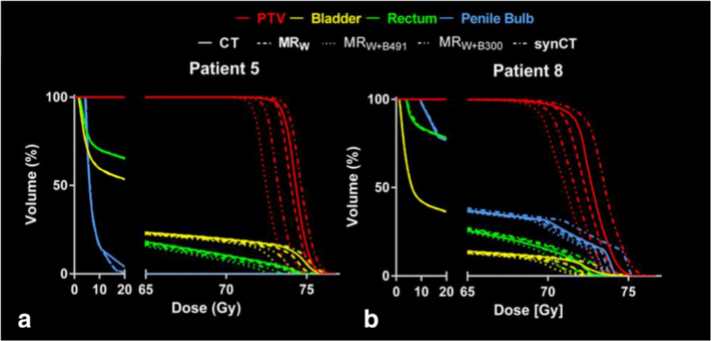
Patient dosimetric results. a Average and b worst case patient DVHs. Good agreement is observed for all treatment plans. SynCT provided closest agreement to CT-SIM results for PTV and bladder, while no significant differences were observed for rectum and penile bulb
